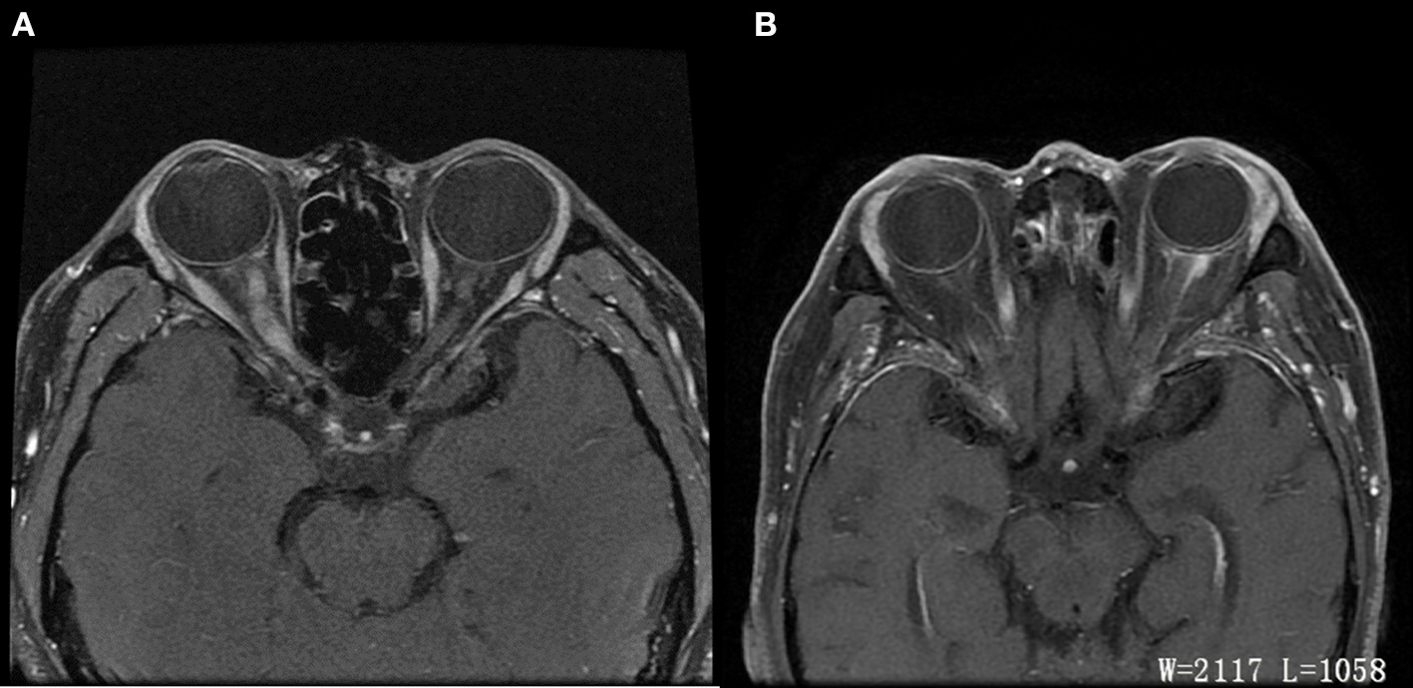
Differences of ON and OPN in MRI. (A) ON: Axial T1 contrast image showed enhancement of right optic nerve. (B) OPN: Axial T1 contrast image showed enhancement of left optic sheath, not enhancement of optic nerve.
